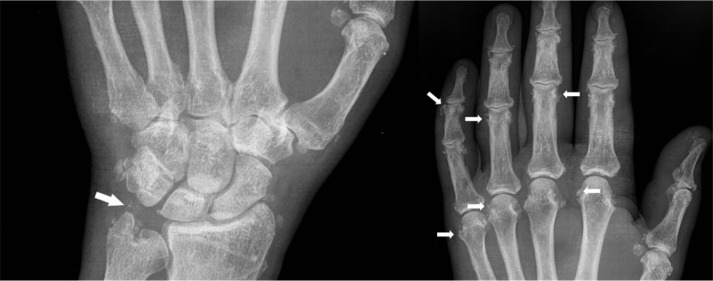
Hand radiographs of patient 1. Left: Chondrocalcinosis of the triangular fibrocartilage of the wrist (arrow). Right: Soft tissue calcifications of the finger joints (arrows).
